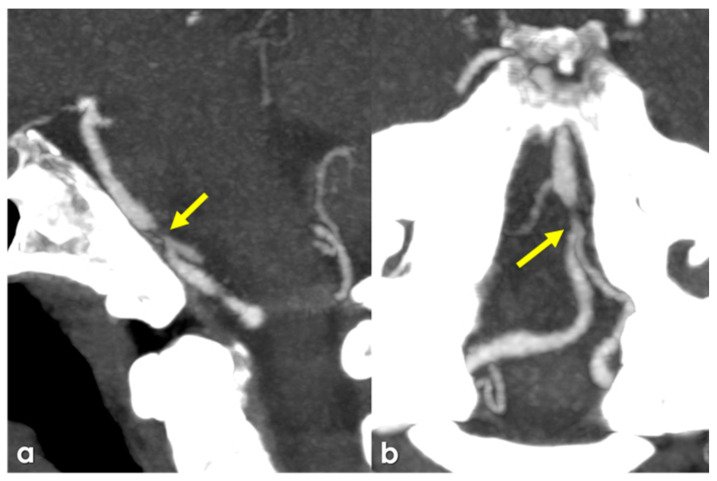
Computed Tomography Angiography (CTA) of a patient with ICAS in the proximal basilar artery (BA), imaged in Multiplanar Reconstruction (MPR) protocol with Maximum Intensity Projections (MPR) processing in sagittal (panel a) and coronal (panel b) view. The yellow arrows point to the BA stenosis.
